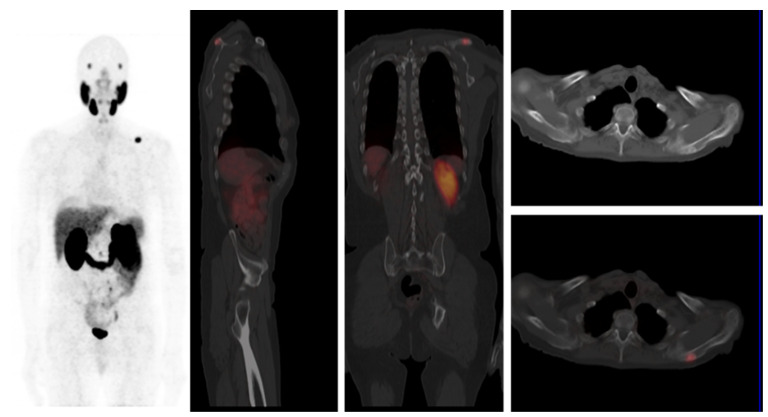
A 68-year-old male known with adenocarcinoma of the prostate, Gleason 4+3. He had radical prostatectomy for local radical therapy. Ga-PSMA-11 PET/CT was obtained at serum PSA level of 3.46 ng/mL to localize the site of disease recurrence. Images obtained from his scan show intense tracer uptake in the left scapula consistent with a solitary skeletal site of extra-pelvic recurrence of prostate cancer. Multiple focal areas of increased tracer accumulation seen in the maximum intensity image (right image) on the left side of the midline in the abdominopelvic area are consistent with urine within the left ureter.
